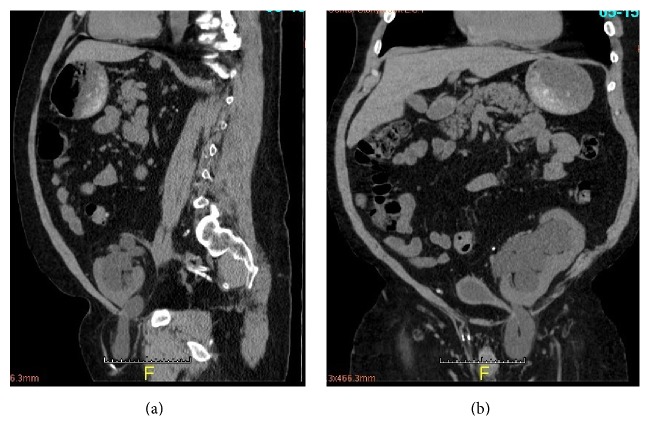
Noncontrast CT abdomen pelvis in sagittal (a) and coronal (b) views revealing transplant ureter within inguinal hernia resulting in hydroureteronephrosis.
